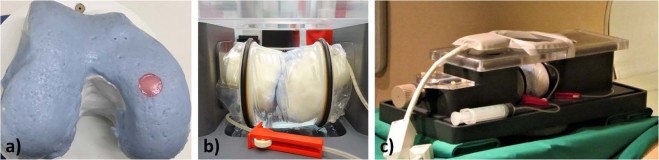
Device for the standardized, displacement-controlled compressive loading of human articular chondral samples. In preparation of this study, an intact right human knee joint had been scanned by CT to obtain the bone contours of femur and tibia which were 3D printed and subsequently covered by wirosil® silicone (as artificial cartilage layer) in its native configuration. (a) At the center of the medial femoral condyle a circular defect (8 mm diameter, 3 mm depth) was created within the wirosil® silicone (blue material) to be filled with native human articular cartilage samples of equal dimensions (red). (b) Loading was brought about by axial displacement of the tibial component (left) against the fixed femoral component (right). The standardized knee was covered by a transparent artificial joint capsule sealed with O-rings and filled with PBS buffer. (c) The assembled and sample-loaded device is displayed within the bore of a 3.0 T MRI system; for imaging, a dual-coil setup was used (attached above and below the knee).
